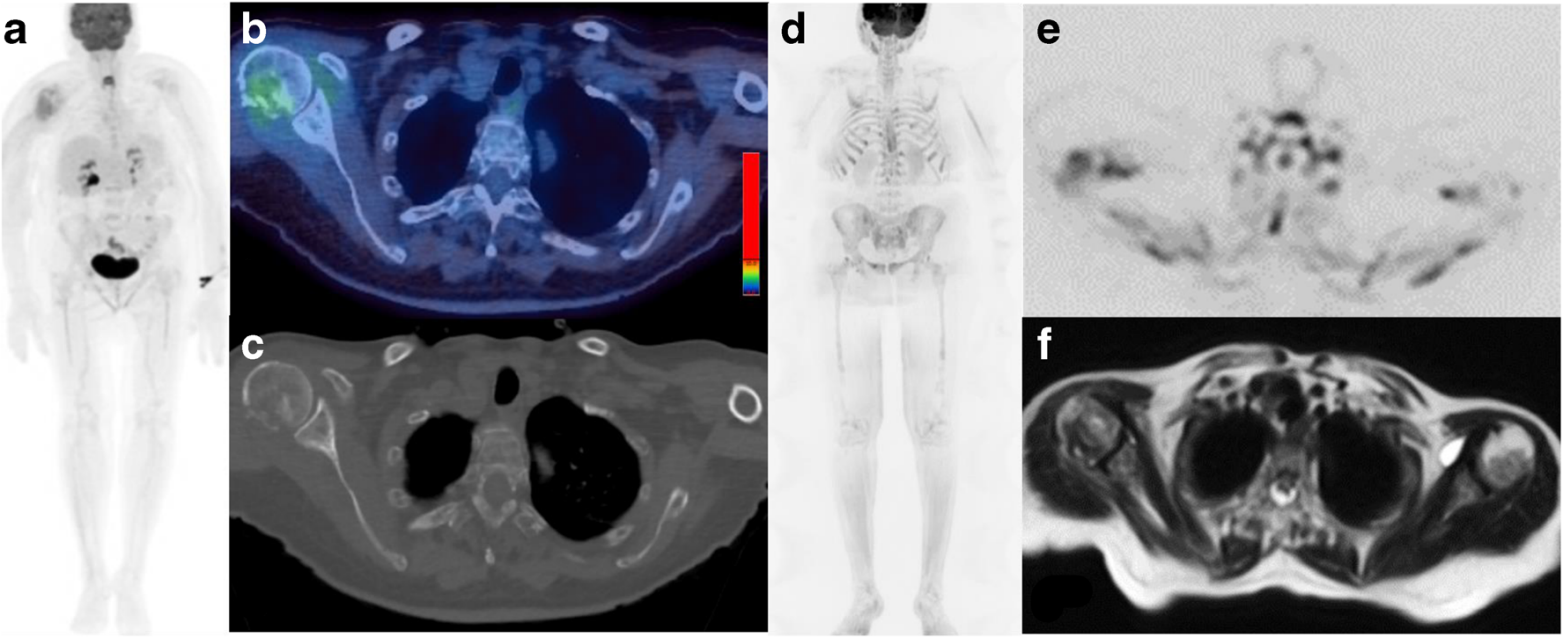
Seventy-six-year-old female with symptomatic myeloma and 80% bone marrow infiltration. 18F-FDG PET/CT (a: Whole body MIP; b: Axial fused image; c: Axial CT image (bone windows)) and WBMRI (d: b900 inverted whole body MIP; e: Axial inverted b900 image; f: Axial T2-weighted image) demonstrates a pathological fracture of the right humeral head as well as more widespread disease with multiple focal lesions (>10) and on both the CT component of the 18F-FDG PET/CT and WBMRI. Beyond the FDG uptake at the fracture site, the background bone marrow: liver FDG uptake ratio was <1.0
